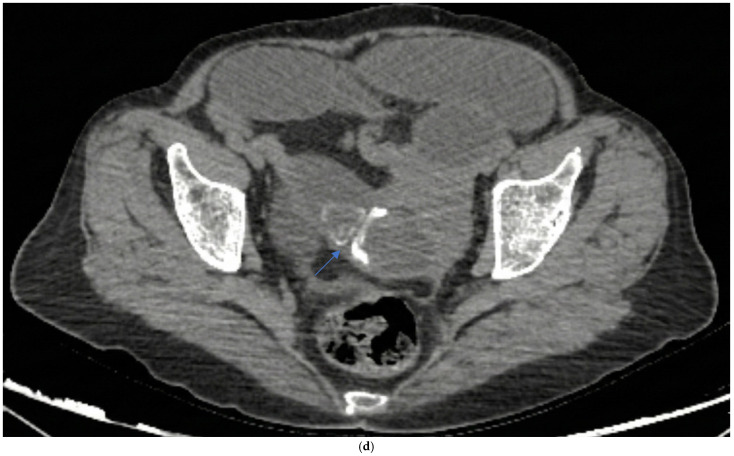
Radiologic images (a) plain X-ray air fluid level and pneumobilia (arrow). (b) Ct-scan bilioenteric fistula and stone (arrow). (c) ileus. (d) terminal ileum gallstone (arrow).
